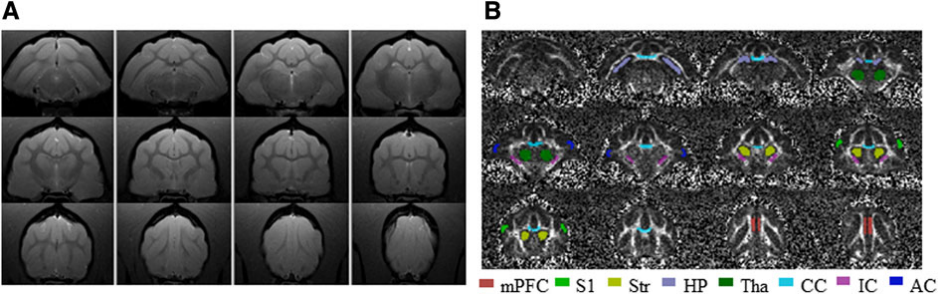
(A) Ferret anatomical MRI. (B) ROIs for DKI and DTI parameters extraction from FA maps. mPFC, medial pre-frontal cortex; S1, primary somatosensory cortex; Str, striatum; HP, hippocampus; Tha, thalamus; CC, corpus callosum; IC, internal capsule; AC, auditory cortex; MRI, magnetic resonance imaging; ROIs, regions of interest; DKI, diffusion kurtosis imaging; DTI, diffusion tensor imaging.
